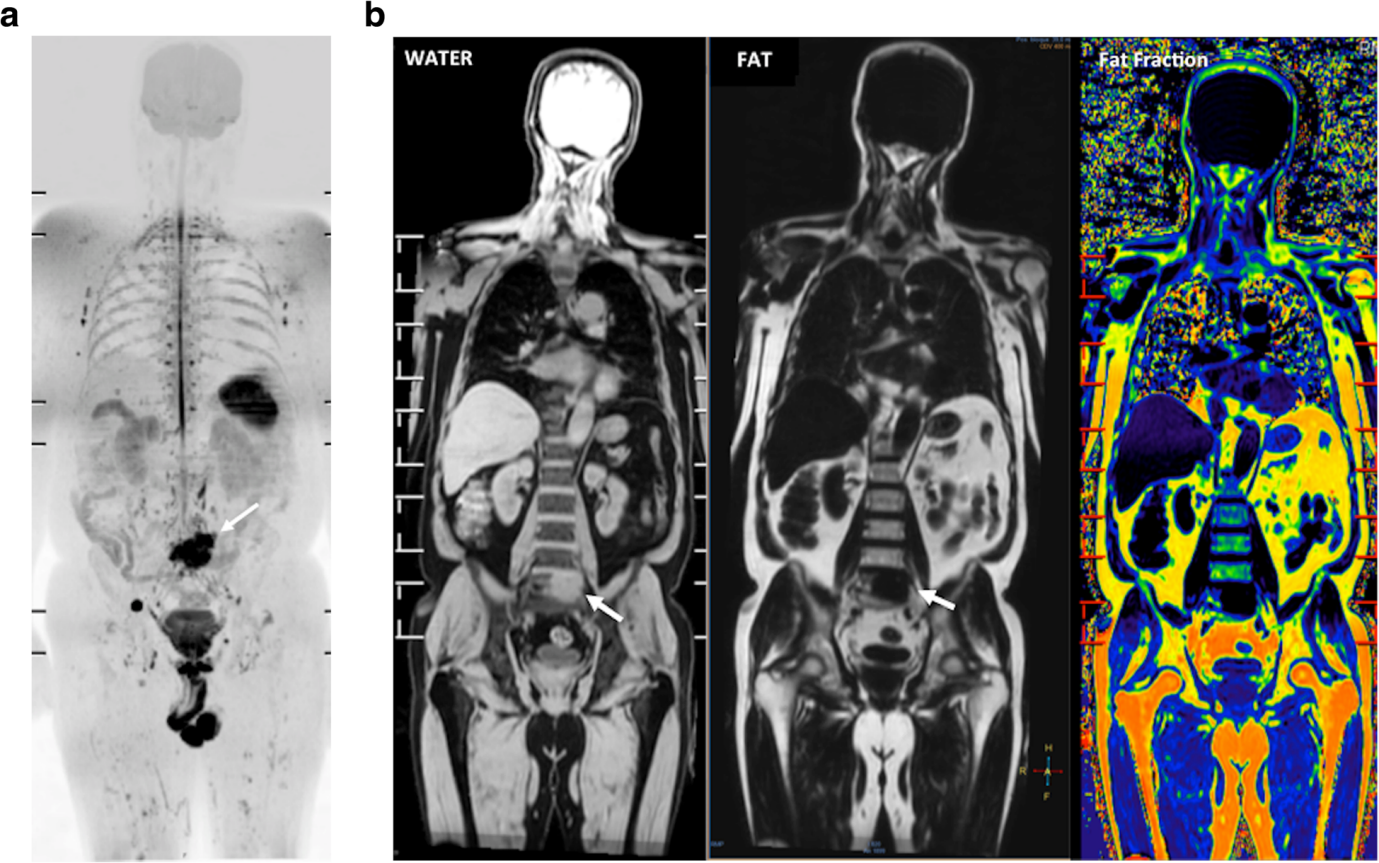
A 68-year-old man with lung adenocarcinoma. a The whole-body diffusion weighted MR image (*b* = 900 s/mm^2^) depicted a metastatic deposit in the sacrum (white arrow). b Coronal reformatted T1W images obtained using the Dixon technique water only (WATER) (left), fat only (FAT) (middle), and fat-fraction in colored scale (right) evidenced not fat content and increased water in the metastatic lesion (white arrow)
